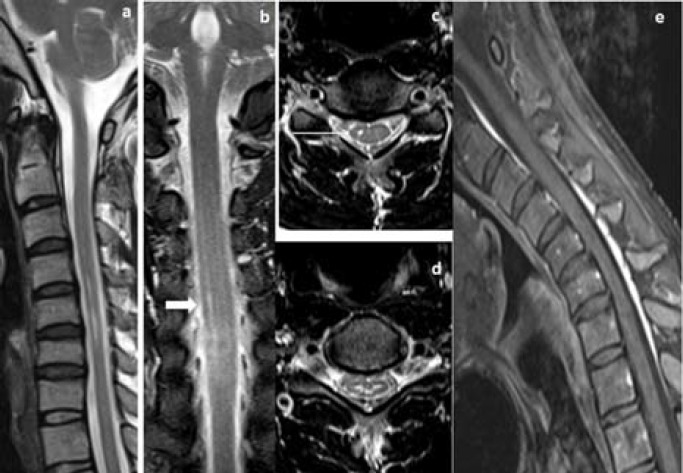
A 17-years old man presented with wasting of bilateral hand muscles. Neural position T2W sagittal and coronal MRI images (a, b) showed segmental hyperintensities in cervical cord extending from C4 to C6 vertebral level, more pronounced in right half of the cord (block arrow), axial T2WI (c, d) showed hyperintensities in bilateral AHC, more pronounced in right AHC and central cord substance (white arrow), post-gadolinium sagittal flexion MRI image (e) showed crescent shaped posterior epidural enhancing lesion with foreword shifting of posterior dural sac
